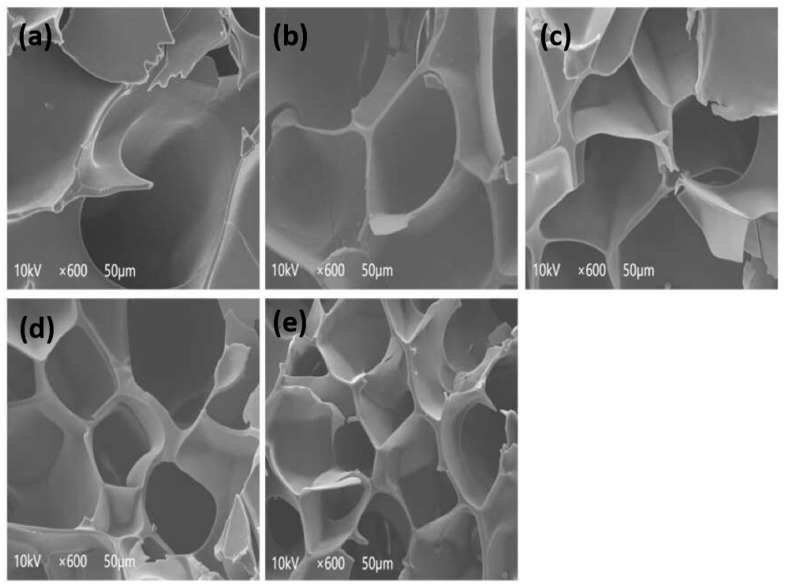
Microstructure of SPI gel (control (a), 400 W ultrasound 60 s (b), 400 W ultrasound 120 s (c), 400 W ultrasound 180 s (d) and 400 W ultrasound 300 s (e), from top to bottom).
